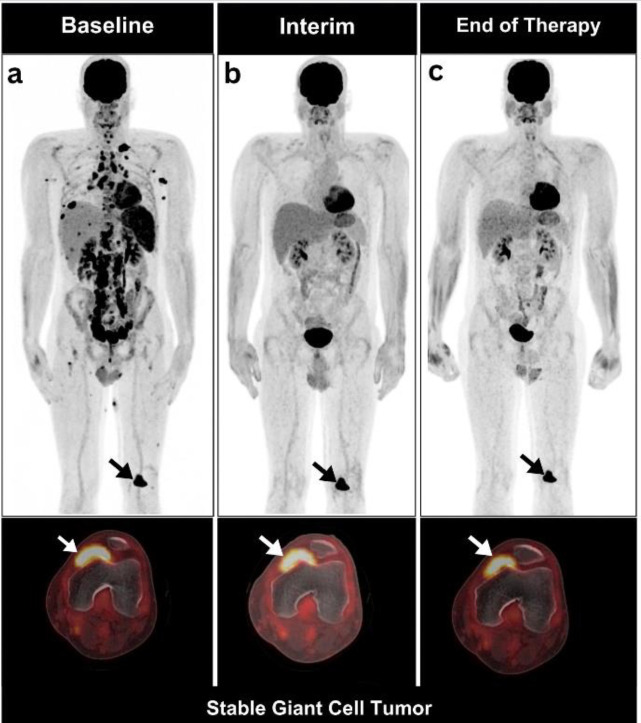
Serial Maximum Intensity Projection images, with their corresponding fused axial PET/CT of the knee (a) performed before chemotherapy, (b) following 3 cycles of chemotherapy (interim), and (c) following the end of therapy demonstrating picture of complete response in Hodgkin Lymphoma but with stable giant cell tumor (arrows)
